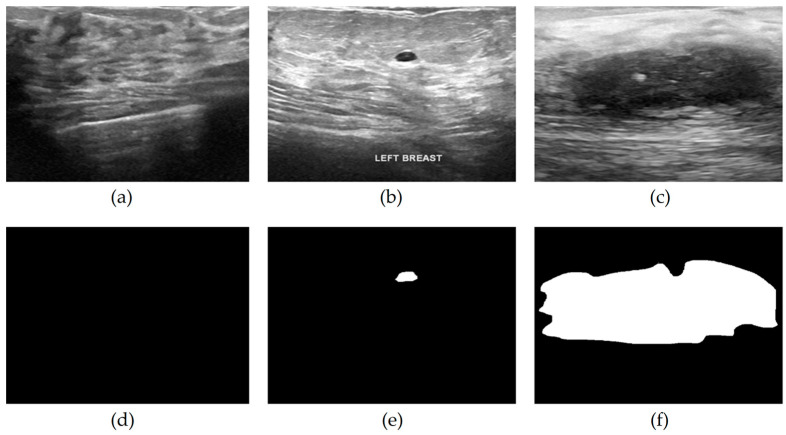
Dataset image samples: (a) normal ultrasound image, (b) benign ultrasound image, (c) malignant ultrasound image, (d) masked image of (a), (e) masked image of (b), (f) masked image of (c).
